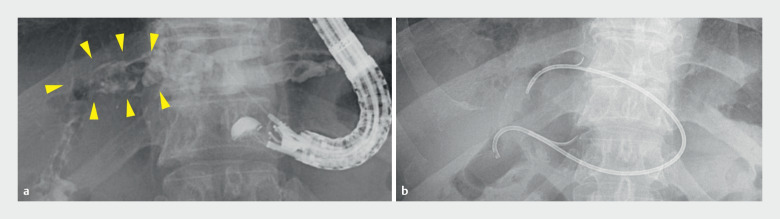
Initial endoscopic ultrasound-guided biliary duct drainage in a 68-year-old man.
